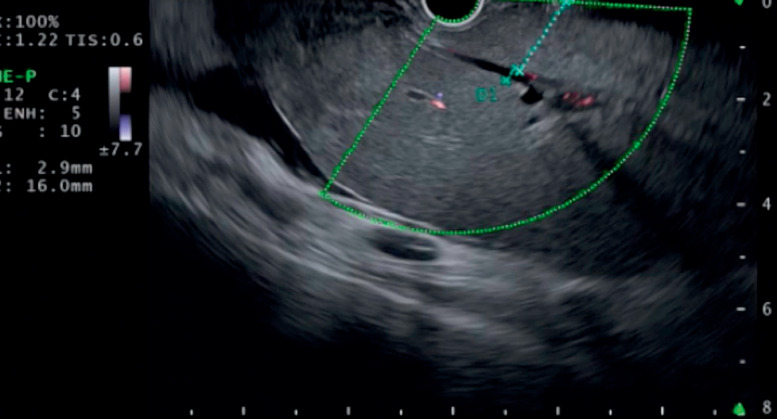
Endoscopic ultrasound image showing that the length of the hepatic parenchyma traversed to reach the puncture site was 16 mm.
